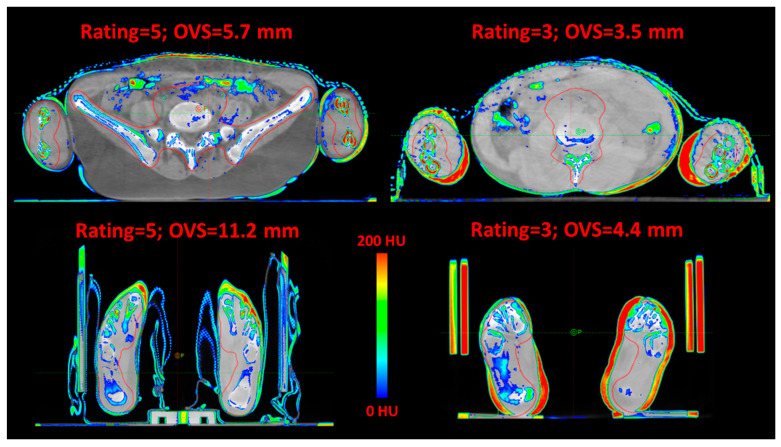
Four representative CBCT-CT matchings of upper and lower extremities. Color wash was used to highlight differences >200 HU. The qualitative rating and OVS are reported. OVS = online vector shift.
